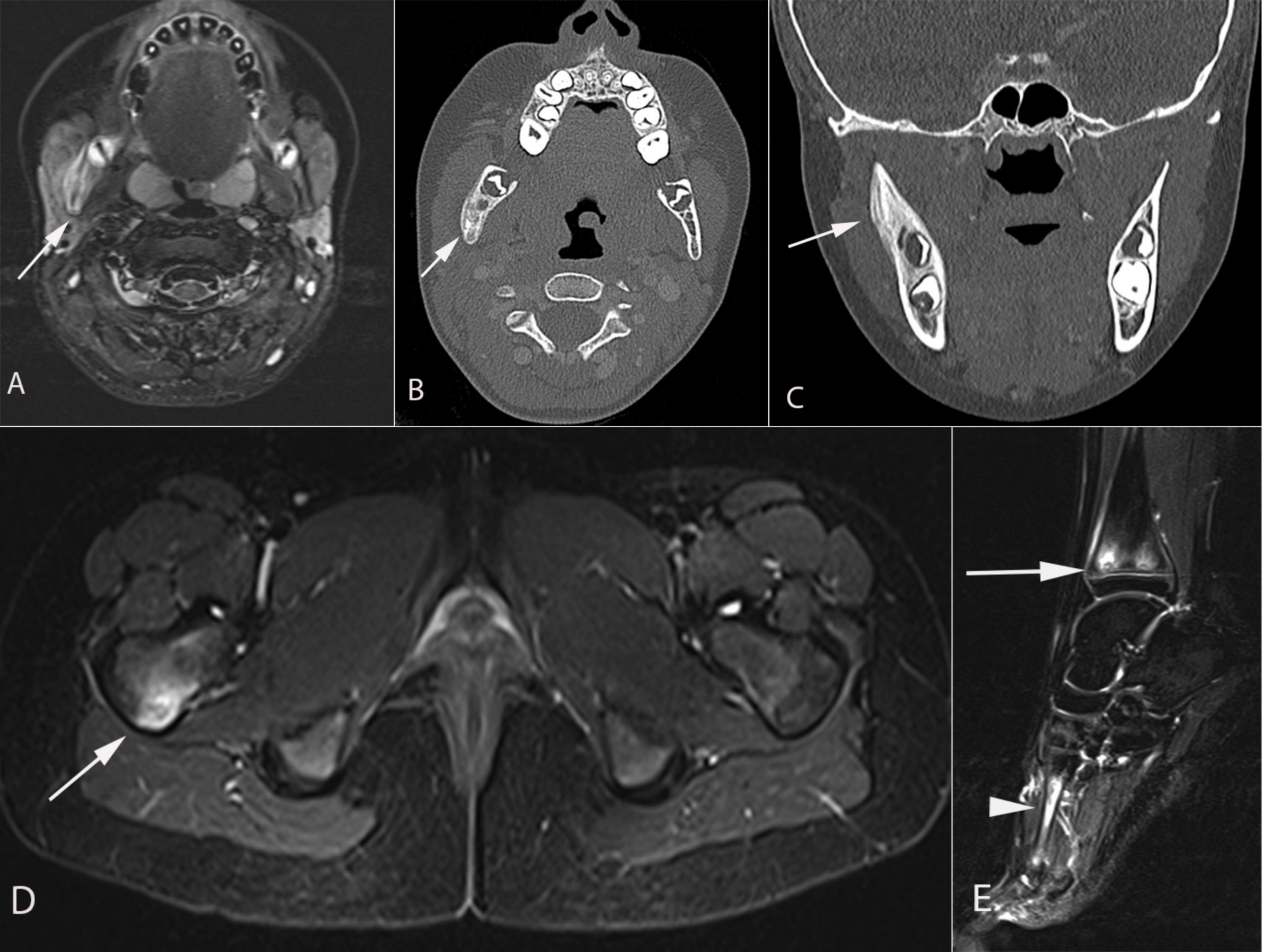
A 9-year-old girl presented with 10 days of right cheek swelling. (A) MRI Axial T2 STIR demonstrated asymmetric enlargement of the right masseter muscle with a heterogeneous hyperintense signal of the right mandibular ramus (arrow) in keeping with bone marrow edema. (B) axial computed tomography and (C) coronal computed tomography images demonstrate smooth periosteal reaction in the right hemi mandible. No associated soft tissue mass or periodontal abscess. Axial (D) and sagittal (E) MRI STIR images demonstrate another focus of hyperintensity in the posterior aspect of the right femoral neck (arrow in D), the distal tibial metaphyses (arrow in e), and the right first (arrowhead in e) and second metatarsals (not shown). Findings are consistent with CRMO following a Whole Body MRI.
